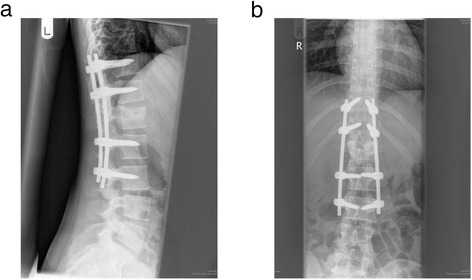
Radiograph of lower thoracic and lumbar vertebrae 12 months after stabilisation and correction a. Lateral b. Anterio-posterior.
